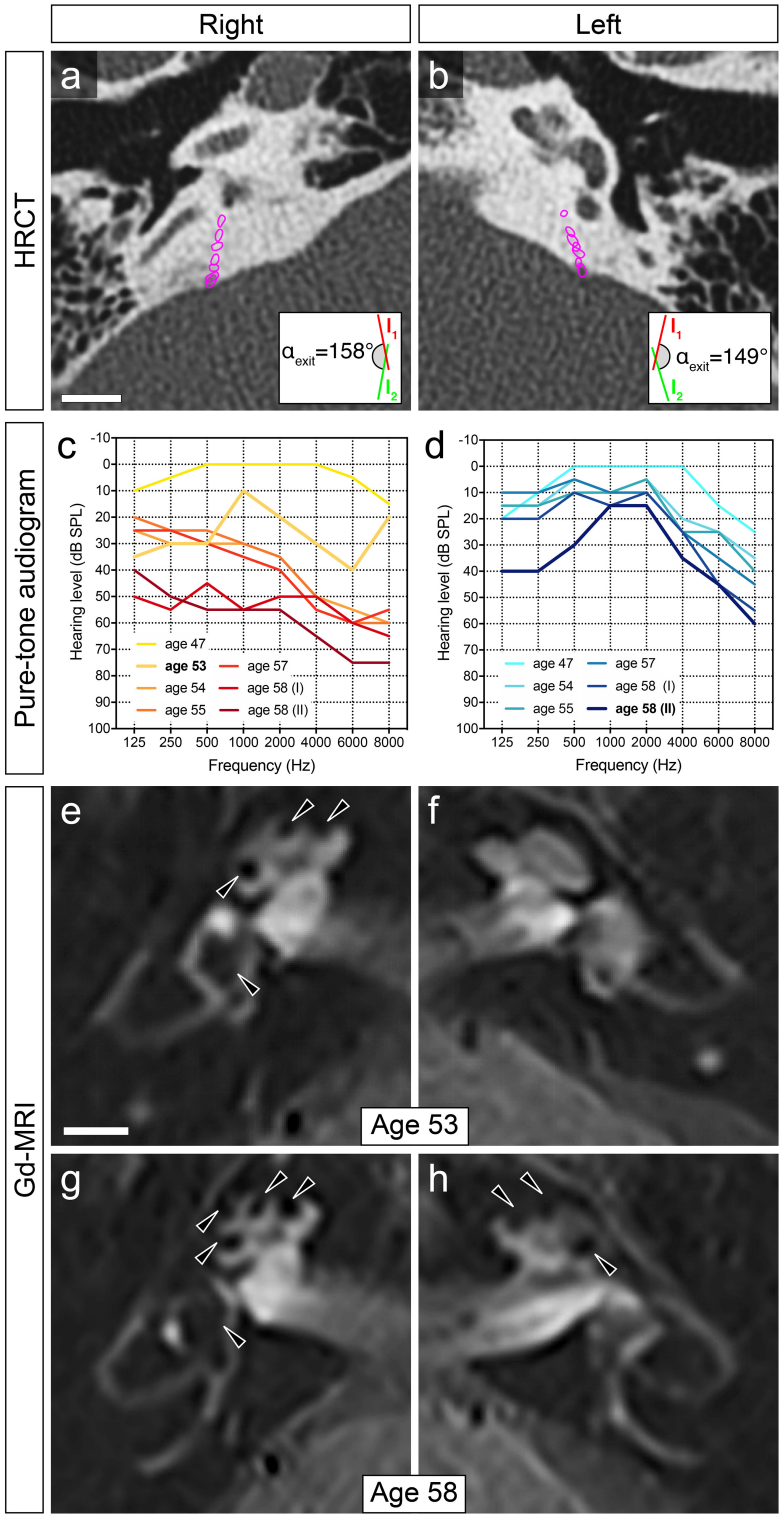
Exemplary clinical case progressing to bilateral disease. (a,b) In this patient, ATVA measurements on temporal bone HRCT images performed at age 53 determined angles (α_exit_) consistent with bilateral ES hypoplasia (2D-reconstructed course of the vestibular aqueduct outlined in magenta). Scale bar: 5 mm. (c,d) Audiogram data (air conduction thresholds) before MD first manifested (age 47), after onset of unilateral (right) MD [ages 53–58 (I)], and shortly after progression to bilateral disease [age 58 (II)]. (e,f) Gd-MRI at the time point of initial MD diagnosis (age 53) demonstrated grade 1 (18) cochleovestibular hydrops (black arrowheads) in the right inner ear. (g,h) Sequential Gd-MRI, after clinical and audiometric data indicated progression to bilateral MD (age 58), demonstrated grade 1 cochleovestibular hydrops in the right and left inner ears. Scale bar: 5 mm.
